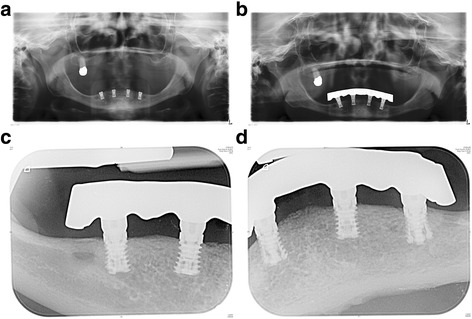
a Postoperative orthopantomogram. b Follow-up orthopantomogram. c Follow-up standard periapical radiogram (implants i42 and i44). d Follow-up standard periapical radiogram (implants i32 and i34)
